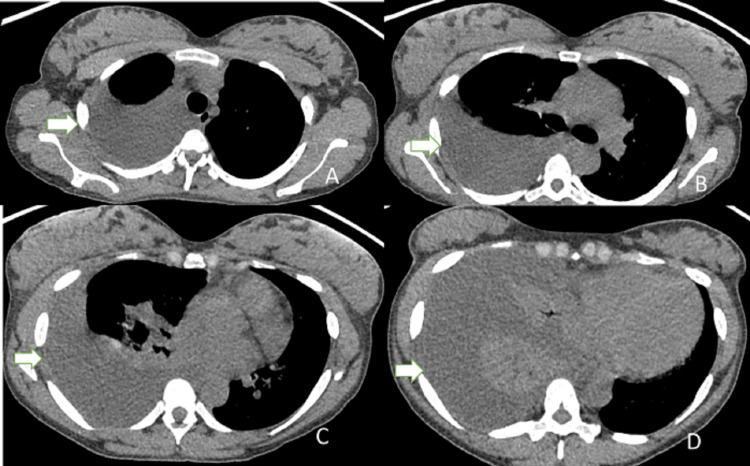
Initial CT of the chest and mediastinal windows Right pleural effusion (white arrows) A. upper lobes; B. carina; C. middle lobe; D. lower lobes
